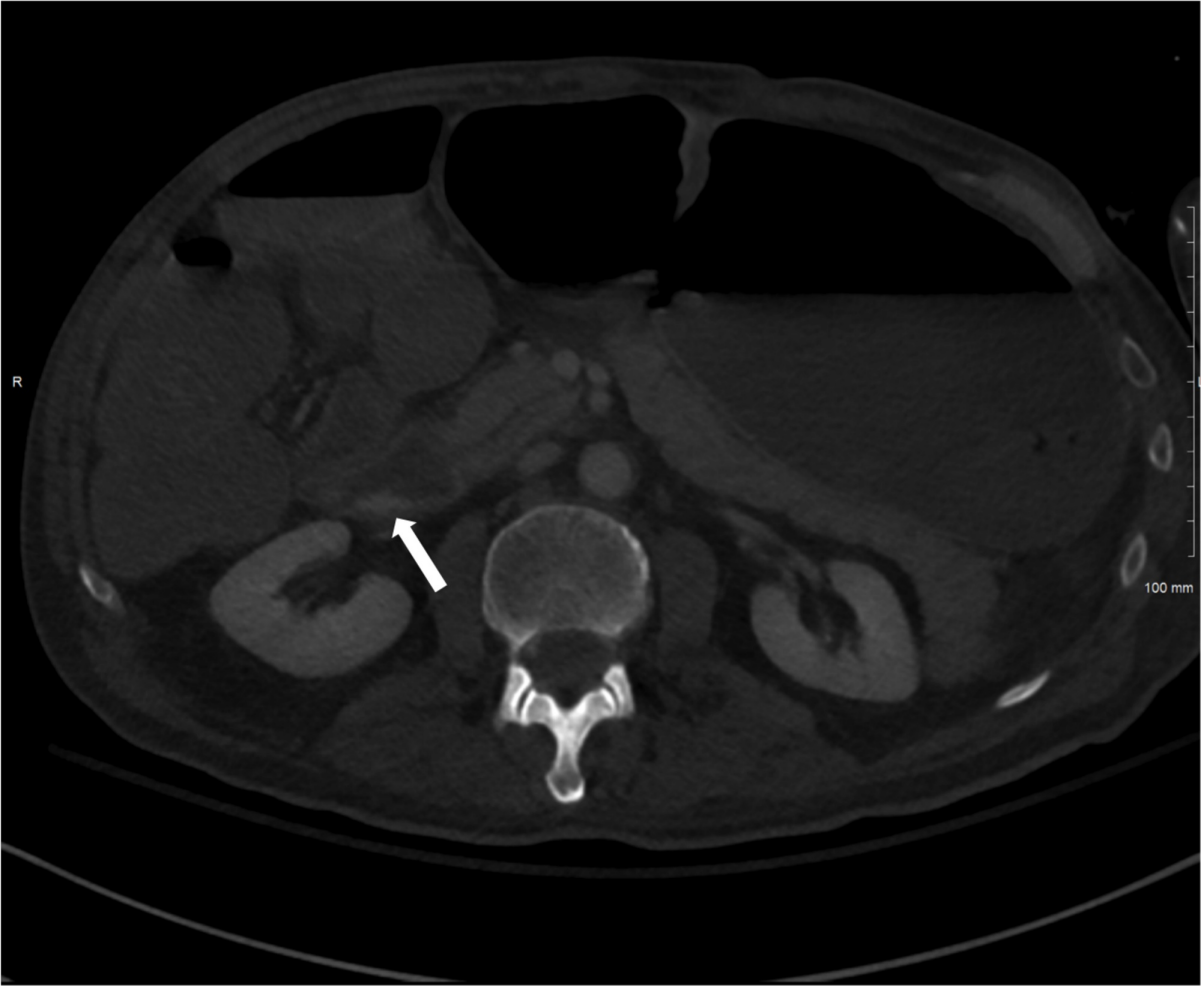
Computed tomography angiography of the abdomen. Axial contrast-enhanced abdominal computed tomography angiography demonstrates active extravasation from the posterior wall of the duodenum (white arrow).
